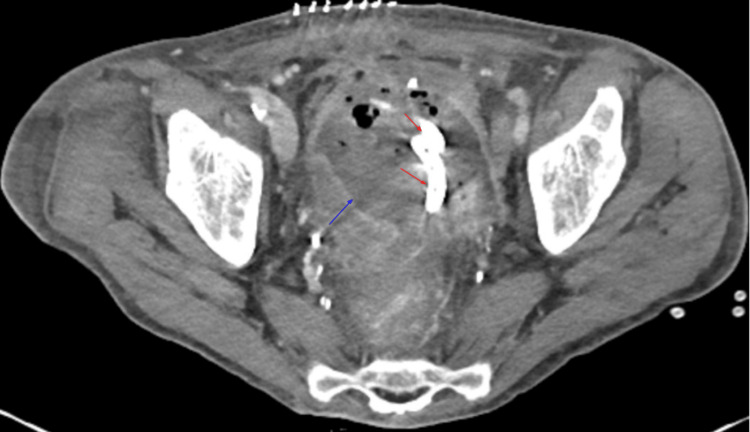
CT abdomen/pelvis in axial view demonstrating decreased size of an associated complex gas and fluid collection with feculent material (blue arrow) and two large caliber drainage catheters in place (red arrows) CT: Computed tomography
